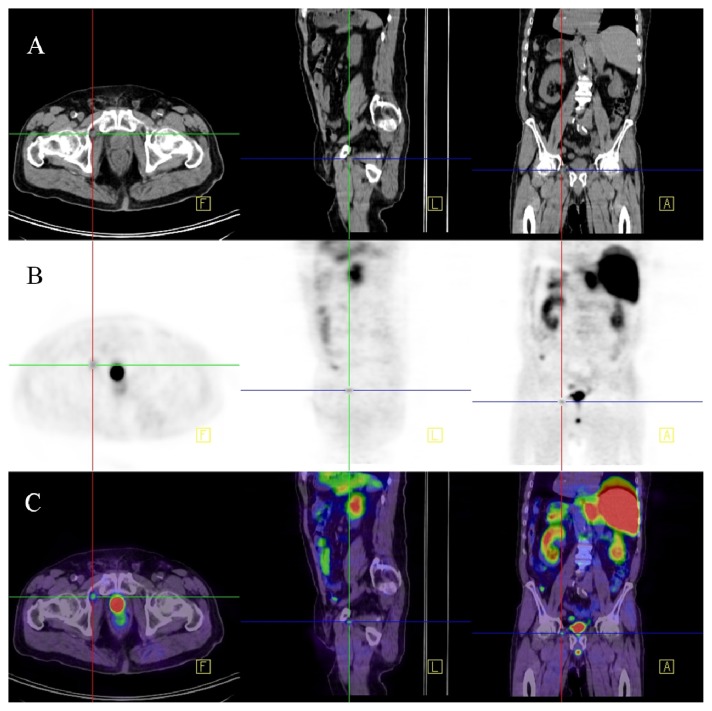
^18^F-choline PET-CT. Patient after radical prostatectomy with slowly increasing PSA (current PSA: 1.5 μg/L). The figure shows series of native CT images (A); PET images 60 min after the administration of ^18^F-choline (B) and fused PET-CT images (C) in transversal, sagittal and coronal views. The PET images show a small hot spot in the right inguinal region with increased isotope uptake. In the PET-CT fused images this spot can be identified as a small right inguinal lymph node highly suspect for a metastasis. (F: Transverse plane; L: Saggital plane; A: Coronal plane).
